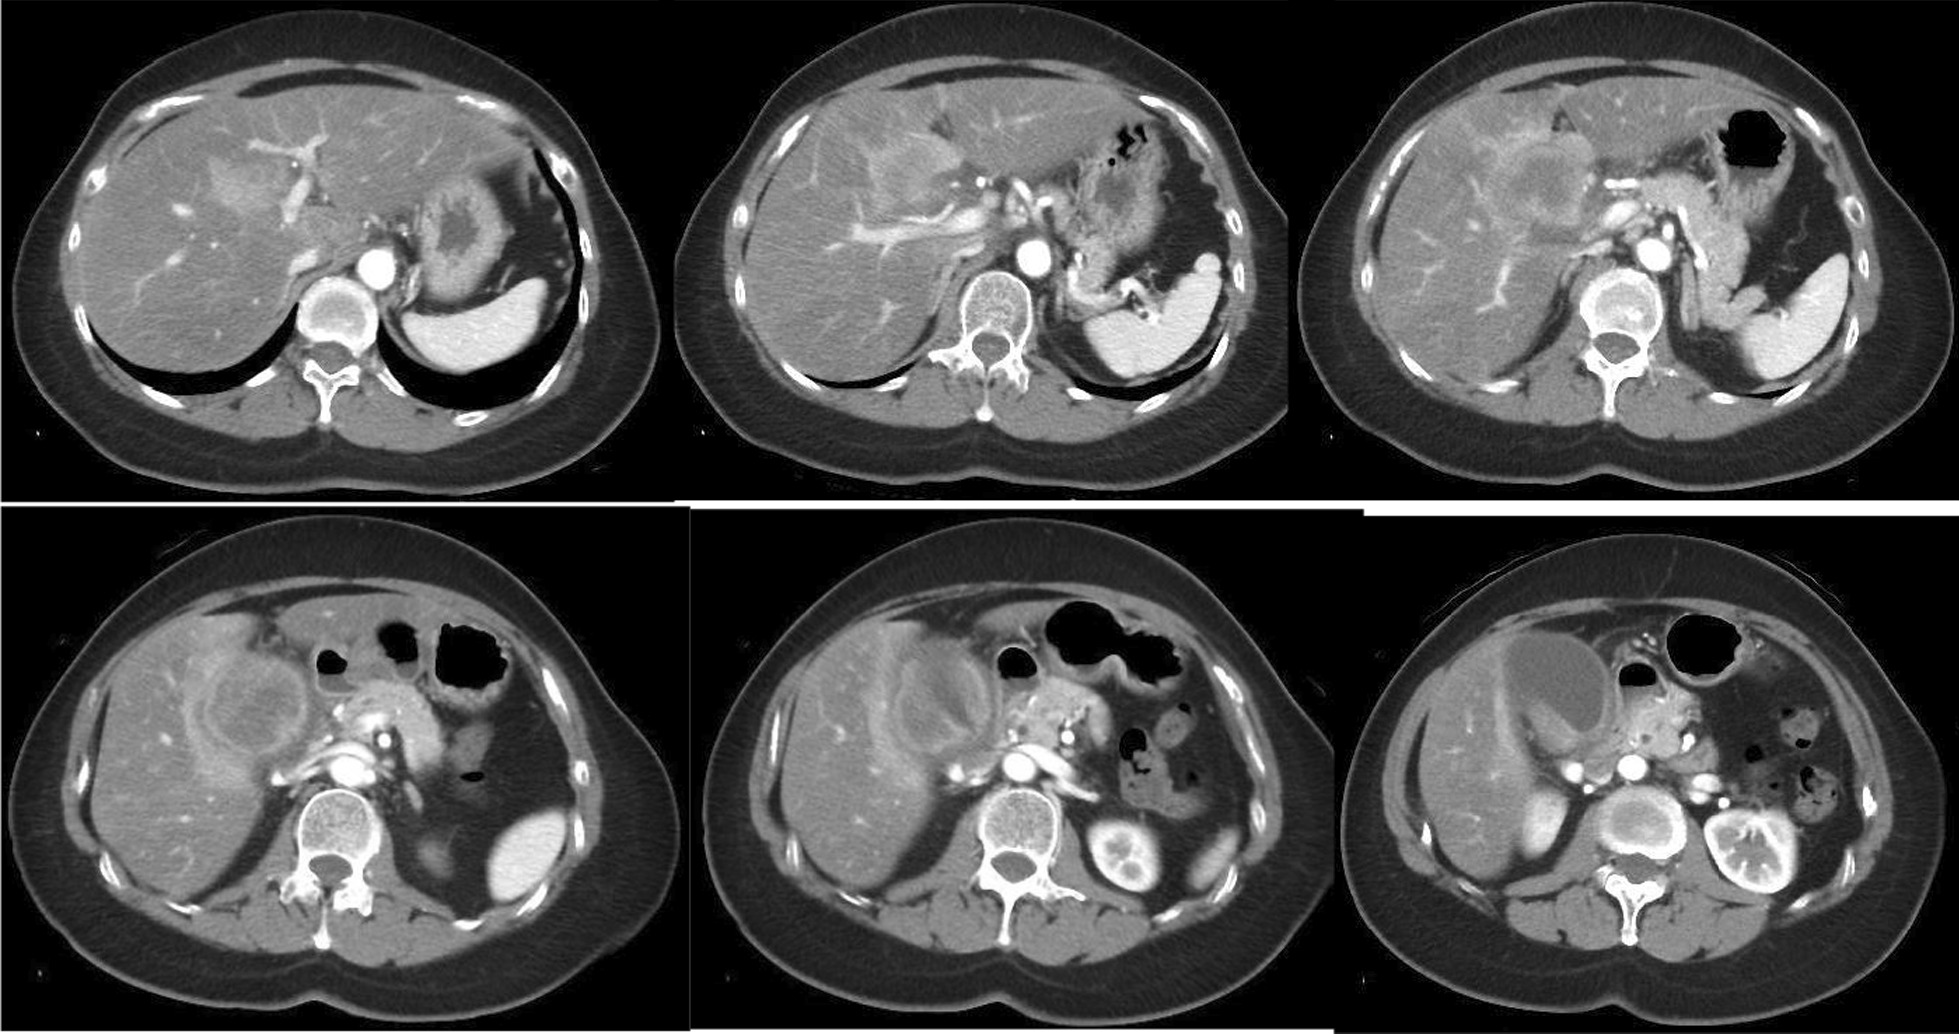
CT revealed a 49 × 47 mm mass at the neck of the gallbladder, with suspected infiltration of the liver and right hepatic artery.
